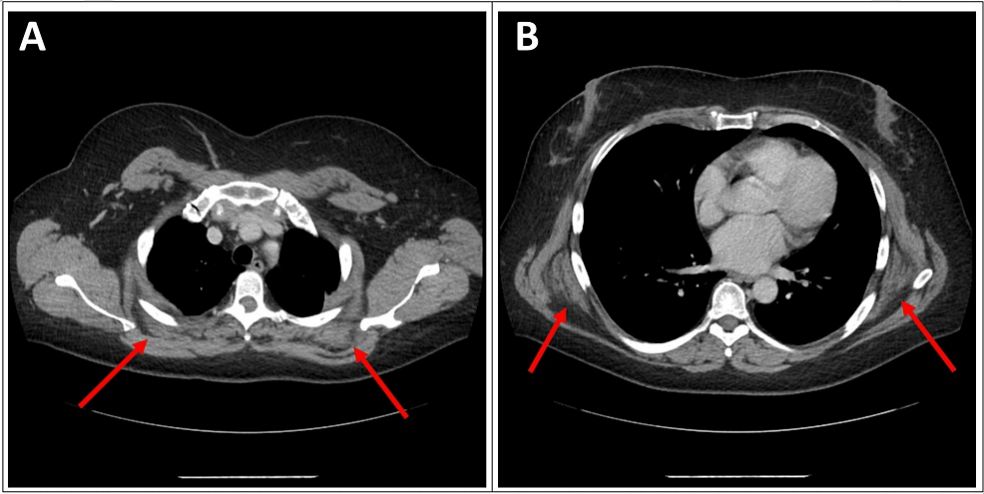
Contrast-enhanced axial plane CT images demonstrate (A) bilateral suprascapular soft tissue density masses, located medial to the medial scapular border and deep to the trapezius muscles and (B) bilateral subscapular masses located deep to the serratus anterior and latissimus dorsi muscles.  The masses contain alternating soft tissue and fat density components.
